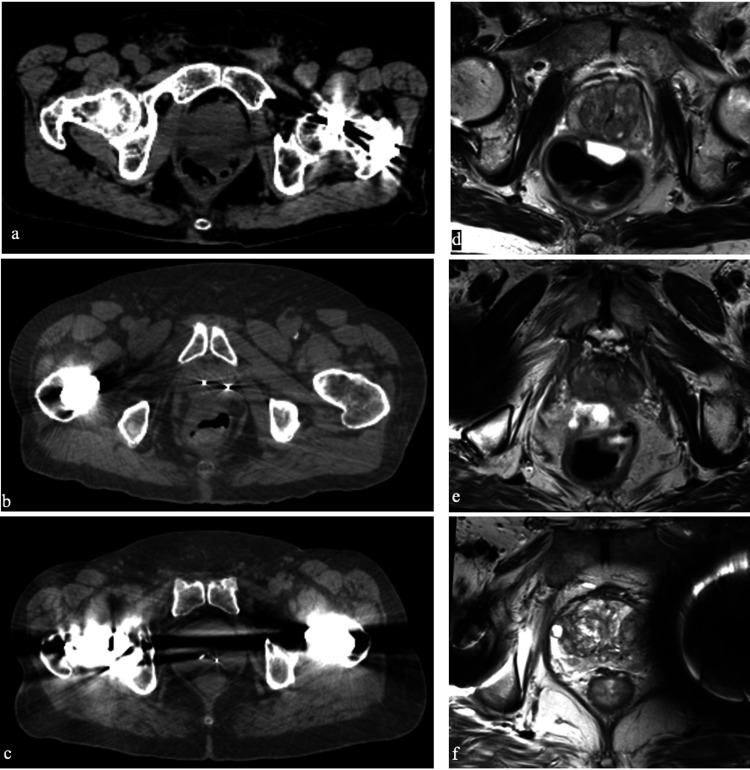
Representative CT and MR treatment planning images, demonstrating: (a) Good-quality CT with unintrusive streak artifact. (b) Adequate-quality CT with unintrusive streak artifact. (c) Suboptimal-quality CT with intrusive streak and banding artifact. (d) High-quality MRI without distortion. (e) Adequate-quality MRI with peri-prosthesis distortion. (f) Suboptimal-quality MRI with intrusive distortion.
